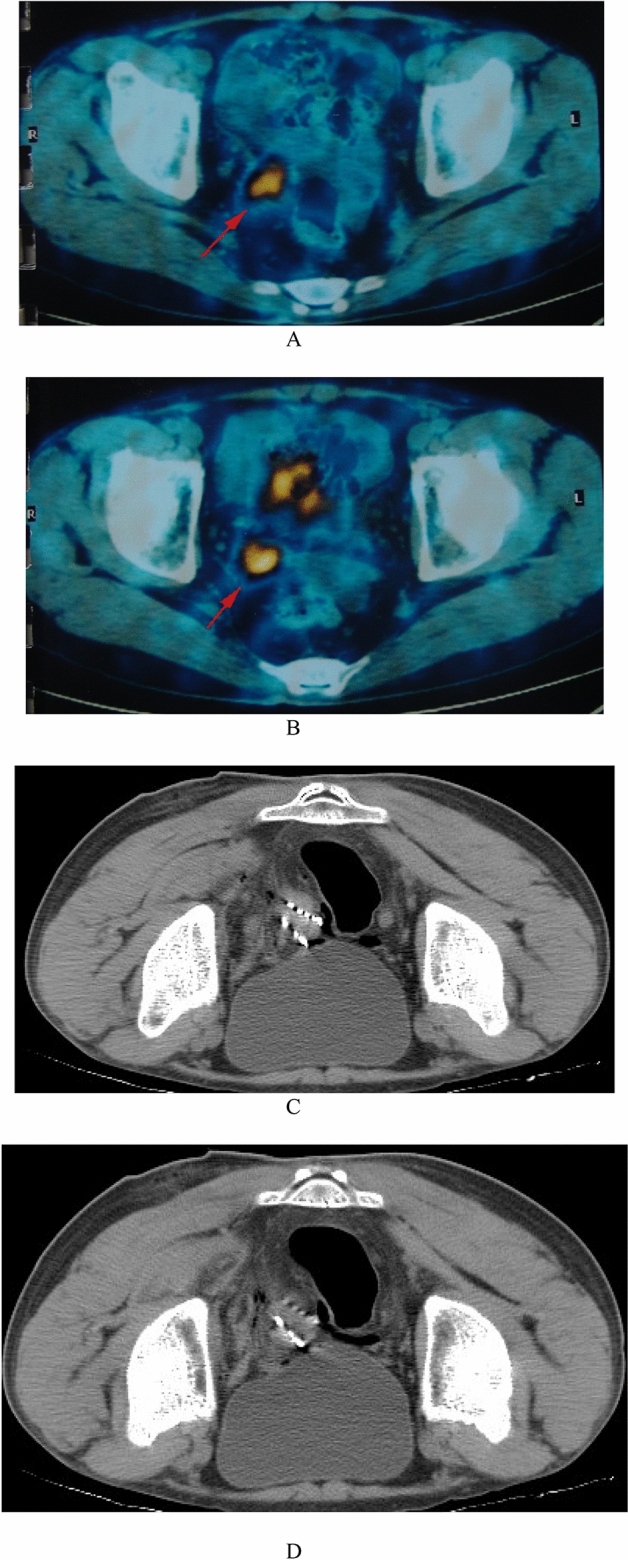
A 35-year-old patient with a tumor of pelvic recurrence after cervical cancer surgery. Tumor dimensions: 20 mm × 2.5 mm × 3.0 mm before treatment. (A,B) The imaging of PET-CT before multimodality minimally invasive treatment showed abnormal radioactivity concentration in the pelvic. (C,D) The imaging of CT after the 125I implantation treatment.
